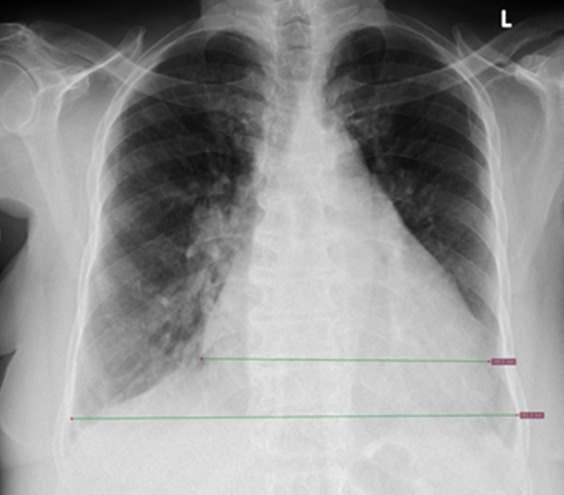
Plain chest radiograph demonstrating transverse thoracic diameter and transverse cardiac diameter measurements in a patient with cardiomegaly
